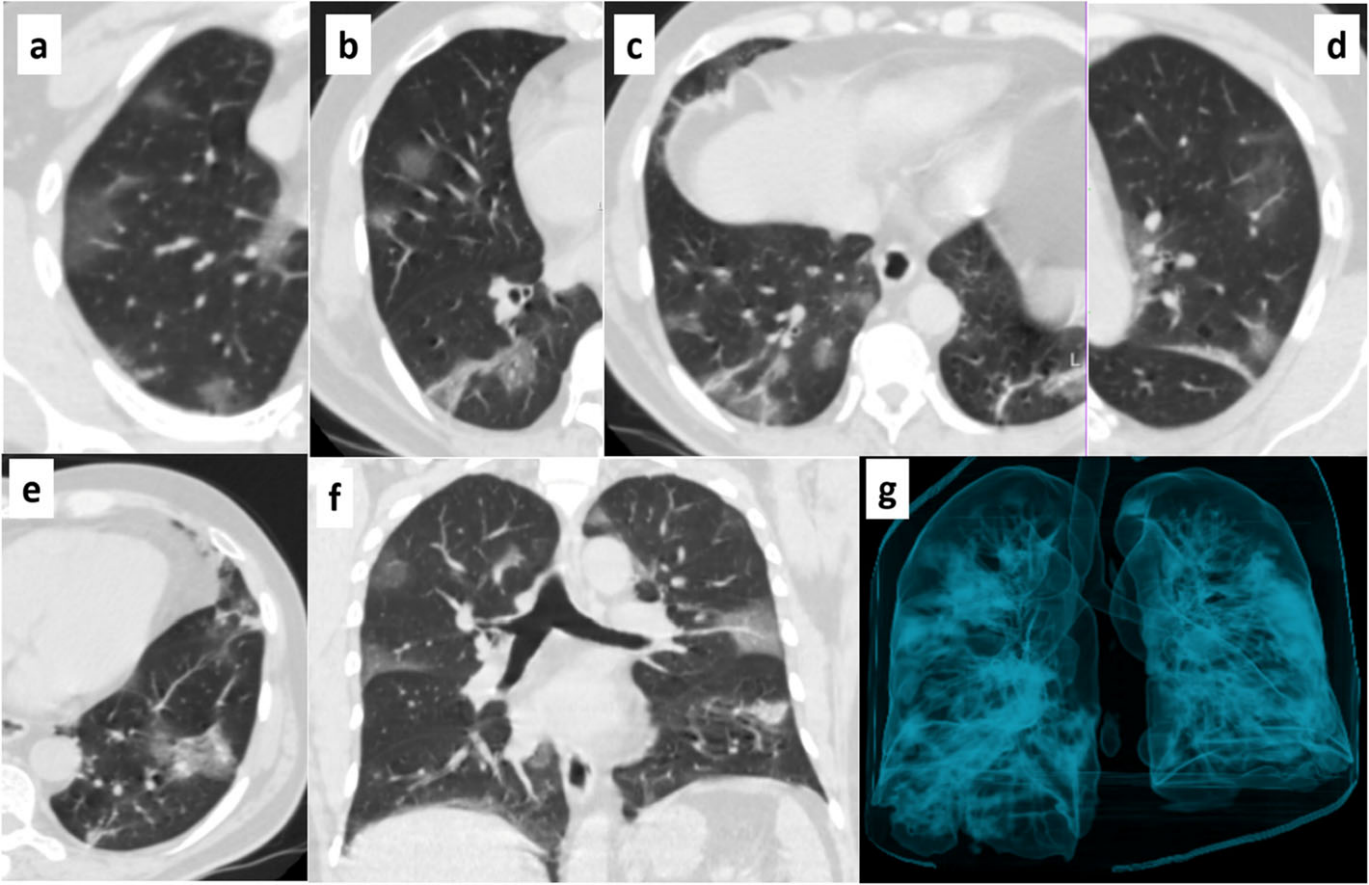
CT severity index of a 51-year-old male patient, progressive stage. a Right upper lobe, score 1. b Right middle lobe, score 1. c Right lower lobe, score 2. d Upper left lobe, score 2. e Left lower lobe, score 2. f Coronal section. g Air volumetric reconstruction. The CT severity index is 8
